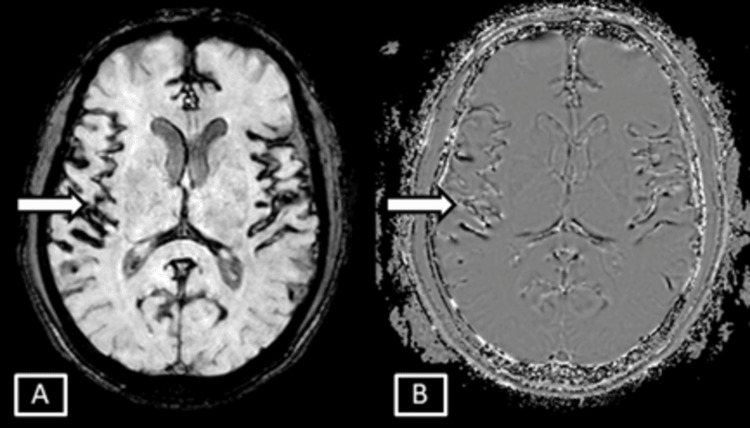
Case 2 MRI brain axial SWI magnitude (A) and phase (B) sequences. Images show symmetrical SWI hypointensites seen involving the sulcal spaces along the cortical surface of bilateral cerebral hemispheres and along the falx represented by the white arrows. SWI: Susceptibility Weighted Imaging
